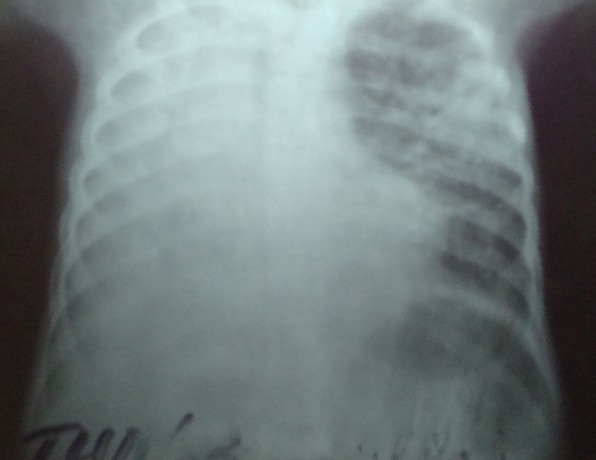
Radiographie du thorax incidence face prise en décubitus dorsal à J1 d'hospitalisation montrant une pleuropneumonie droite ainsi qu'un foyer lobaire supérieur gauche
